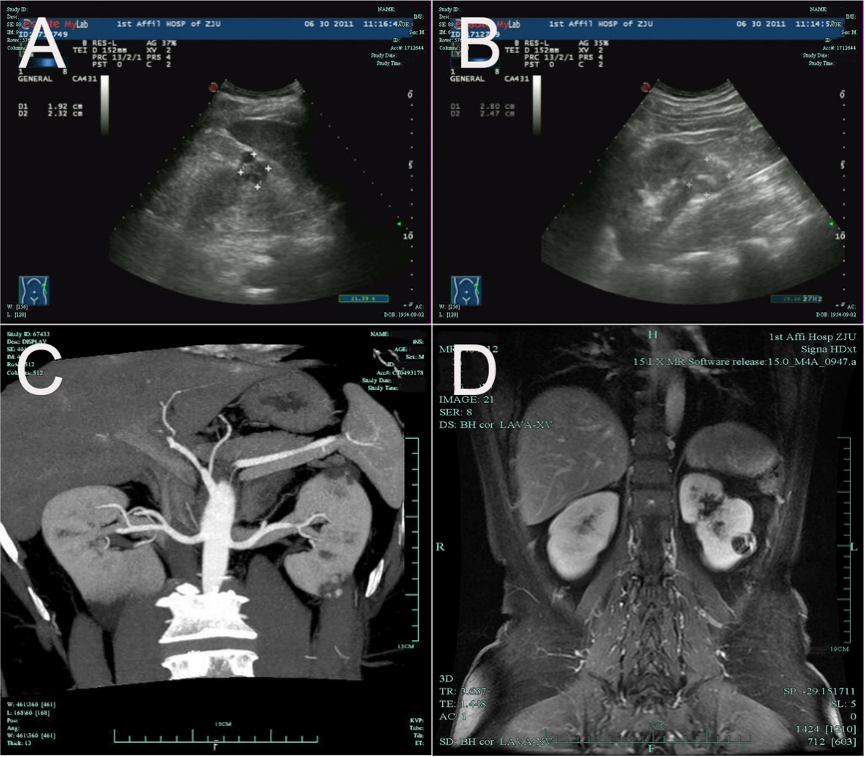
Ultrasound, computed tomography, and magnetic resonance images. A) Ultrasound: Upper pole complex cystic renal mass (patient 2). B) Ultrasound: Lower pole renal solid mass (patient 2). C) Computed tomography: Lower pole renal mass with a concurrent cyst in the upper pole (patient 2). D) T1-weighted magnetic resonance imaging: Complex cystic mass with thick and irregular enhancing cyst walls in the middle part of the kidney.
